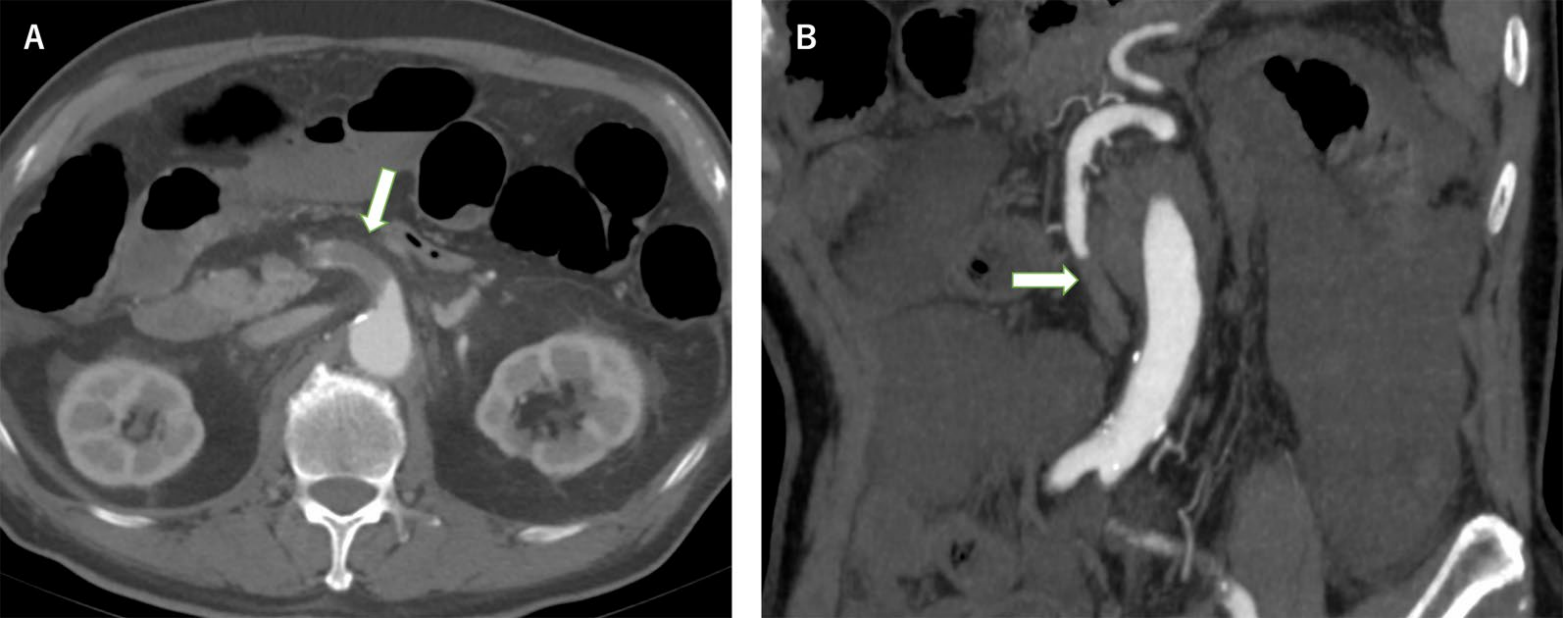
Contrast-enhanced computed tomography scanning revealed total occlusion of the superior mesenteric artery (arrows) (A Case 1, B Case 2)
